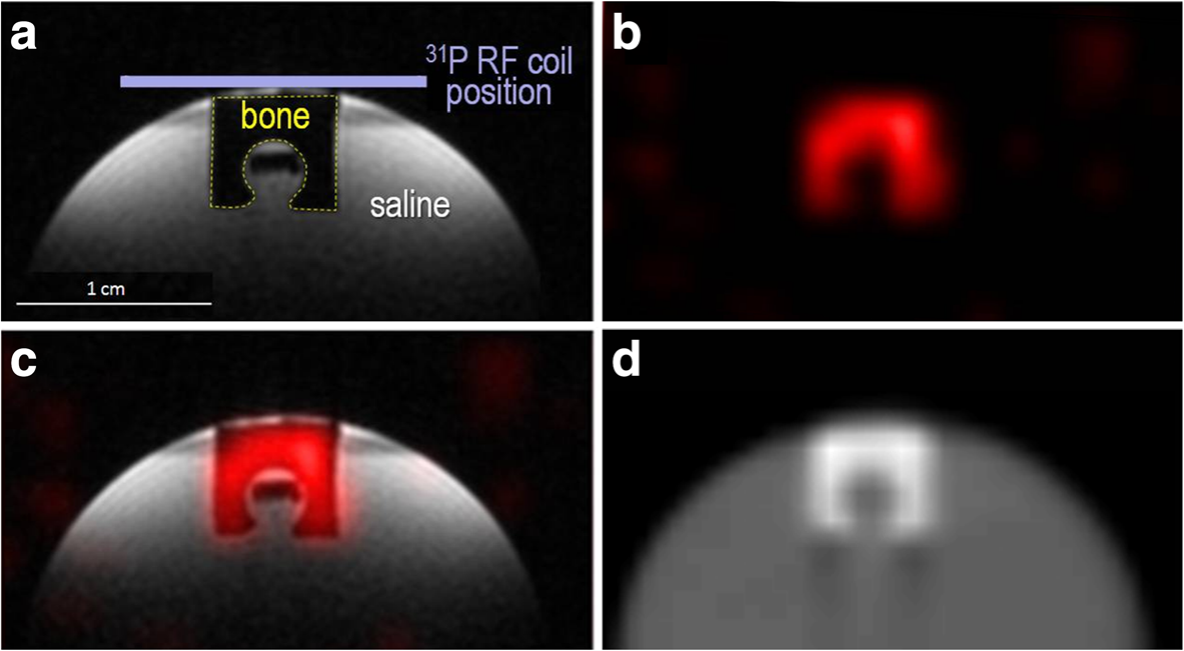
MRI and CT images acquired on the cortical bone sample. ^1^H anatomical MRI (a), ^31^P-ZTE MRI (b), fusion (c), and low-resolution CT (d). Note that the rectangular structure in hyposignal located in the center of the inverted U-shape bone in a is a piece of plastic used to position the bone sample on top of the tube
